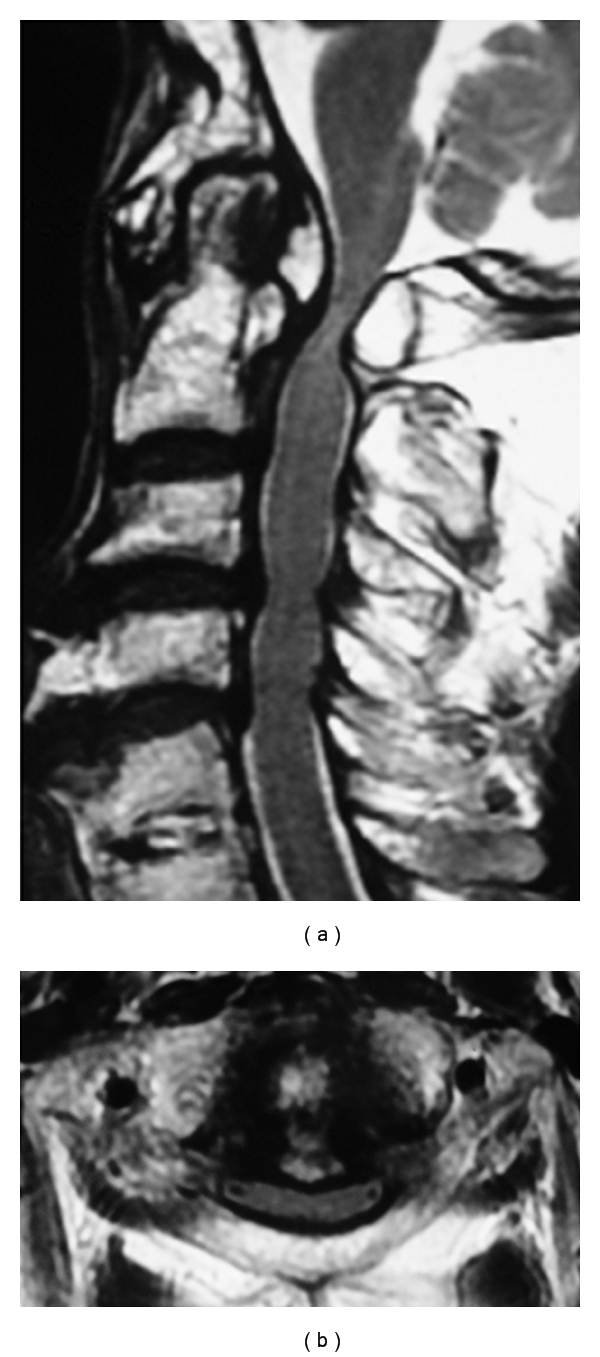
Preoperative MRI on T2-weighted image. (a) Sagittal plane. The spinal cord is compressed at the level of C1, C3-4, and C4-5, with high intensities within the spinal cord at C1 level. (b) Axial plane. The spinal cord is compressed by the mass behind dens and posterior arch of the atlas.
